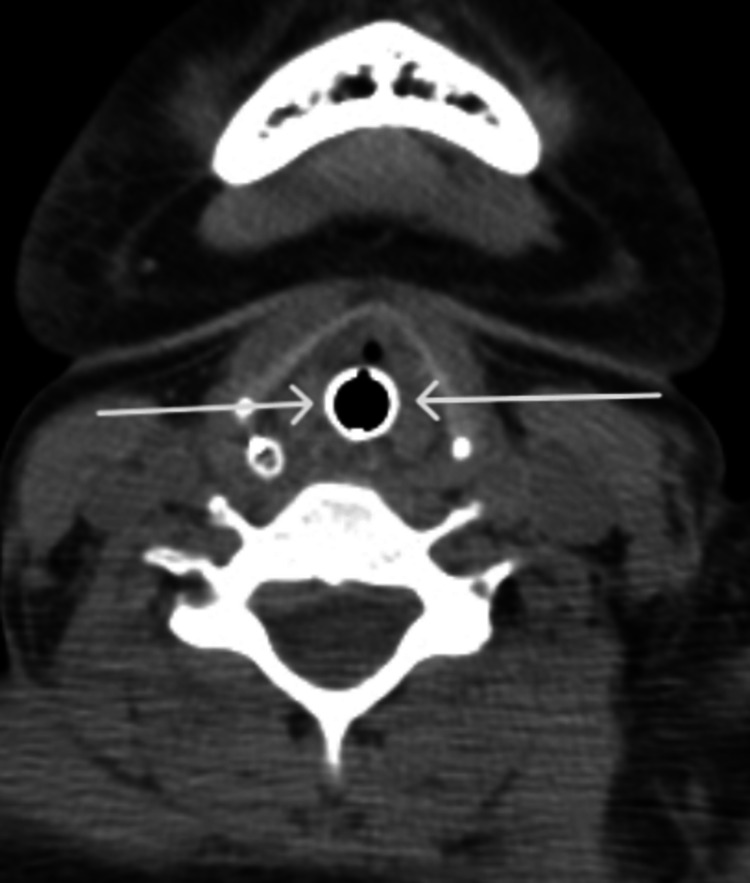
CT scan of the neck (axial section) White arrows show laryngeal edema at the level of true vocal cords.
